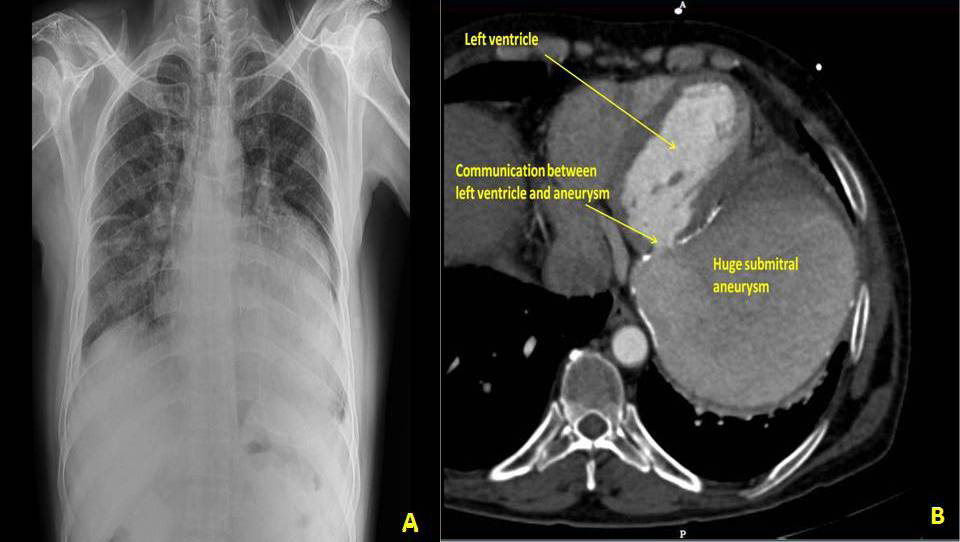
(A) Chest roentgenogram showing calcified cystic lesion in the left hilar region; (B) Computed tomography showing communication between left ventricle and submitral aneurysm.
